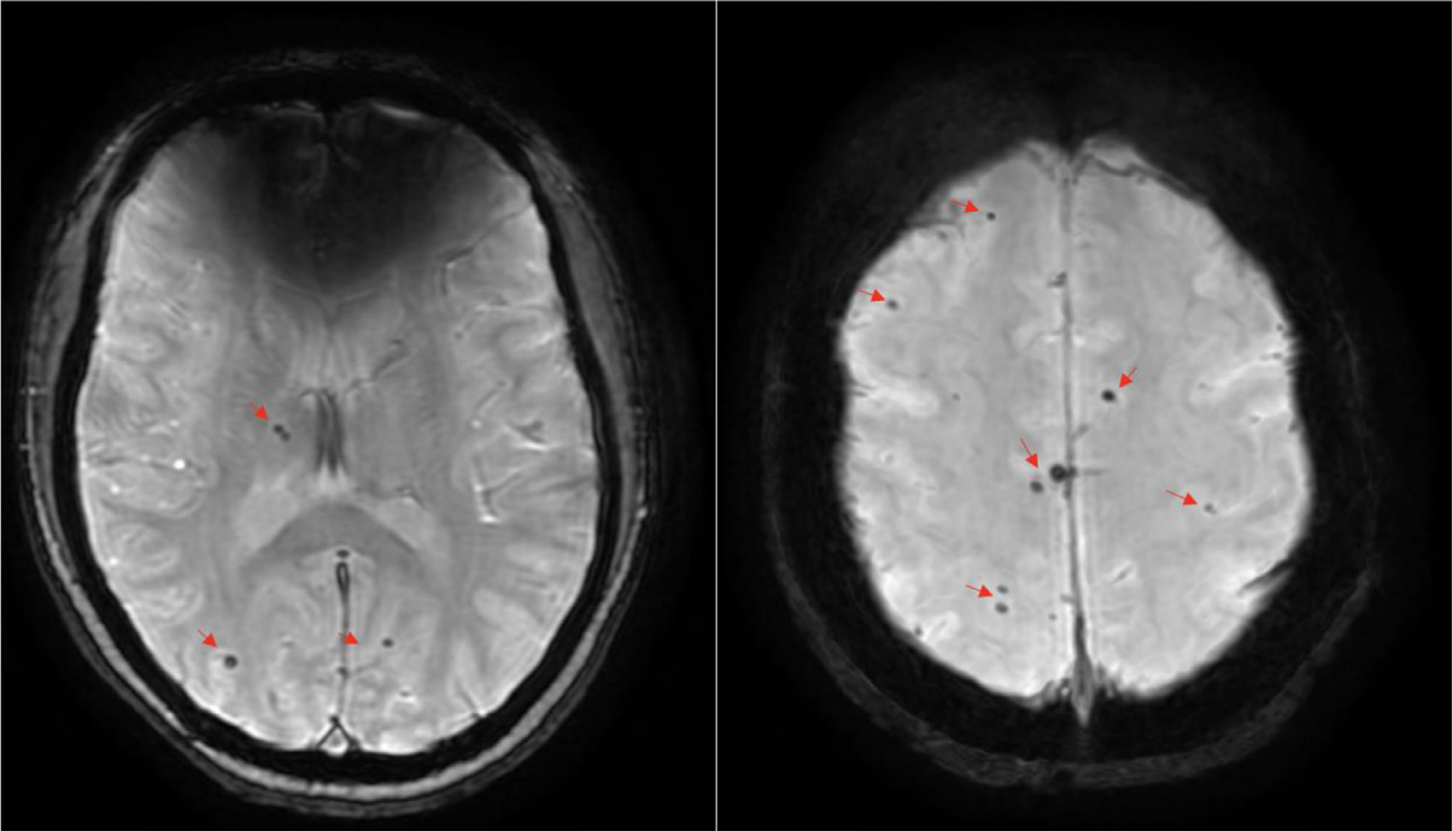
Axial MRI SWAN Sequence (left) and Multiplanar reconstruction (right) showing multiple rounded dark areas in the cerebral hemispheres [Red arrows] corresponding to areas of calcification demonstrated on CT images (Fig 1.). Lesions are noted throughout the cerebral white matter, the thalamus, and the basal ganglia. No other intracranial abnormalities are seen. These findings are consistent with neurocysticercosis.
